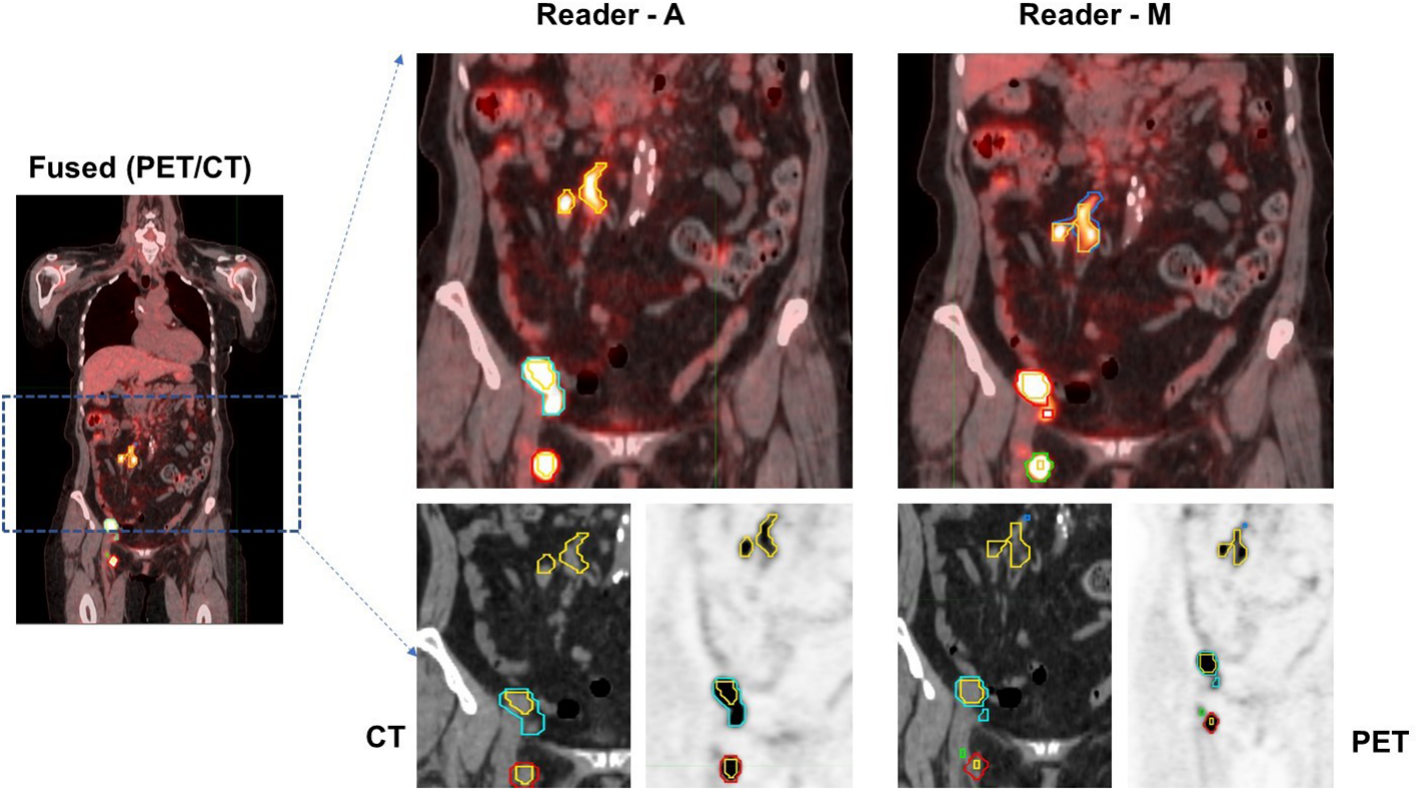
Patients image scan (PET/CT) with lesion delineation shown on a representative slice. Multiple boundaries for a lesion indicate original lesion segmentation (reader M and reader A) and corresponding 41% cut-point (inner boundary for a lesion). Lesions with overlapping regions (original and 41% cut-point) will show one boundary. In the example case, using Reader M’s approach, MTV was estimated to be 28.07 ml (TLG: 328.93 SUV*ml) while Reader A’s approach estimated MTV was 23.25 ml (TLG: 315.25 SUV*ml), with a difference of 17.2% in their MTV estimates.
